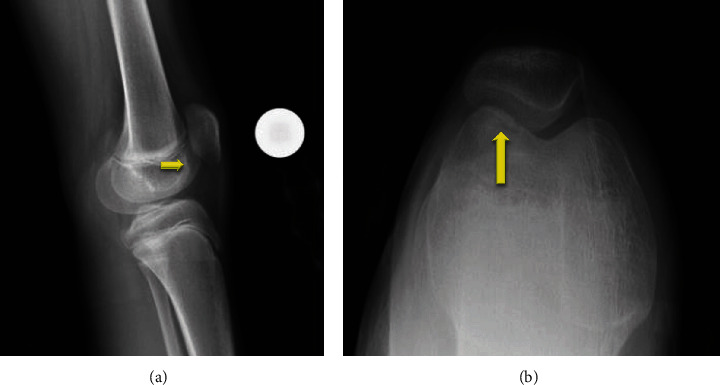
Follow up X-rays of patient with trochlear osteochondritis dissecans (OCD) showing bony healing on (a) lateral and (b) sunrise views of the left knee.
